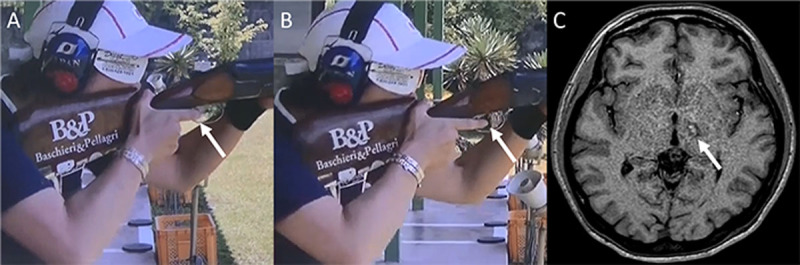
Moment of triggering and postoperative MRI. Distal interphalangeal joint of the triggering finger (arrow) flexed at pre-symptomatic condition (A). 2 months after the onset of dystonia, distal interphalangeal joint of the triggering finger remained extended due to dystonia at the moment of triggering (B). T1-weighted head MRI on the day of the surgery showing coagulated lesion (arrow) on the left thalamus (C).
